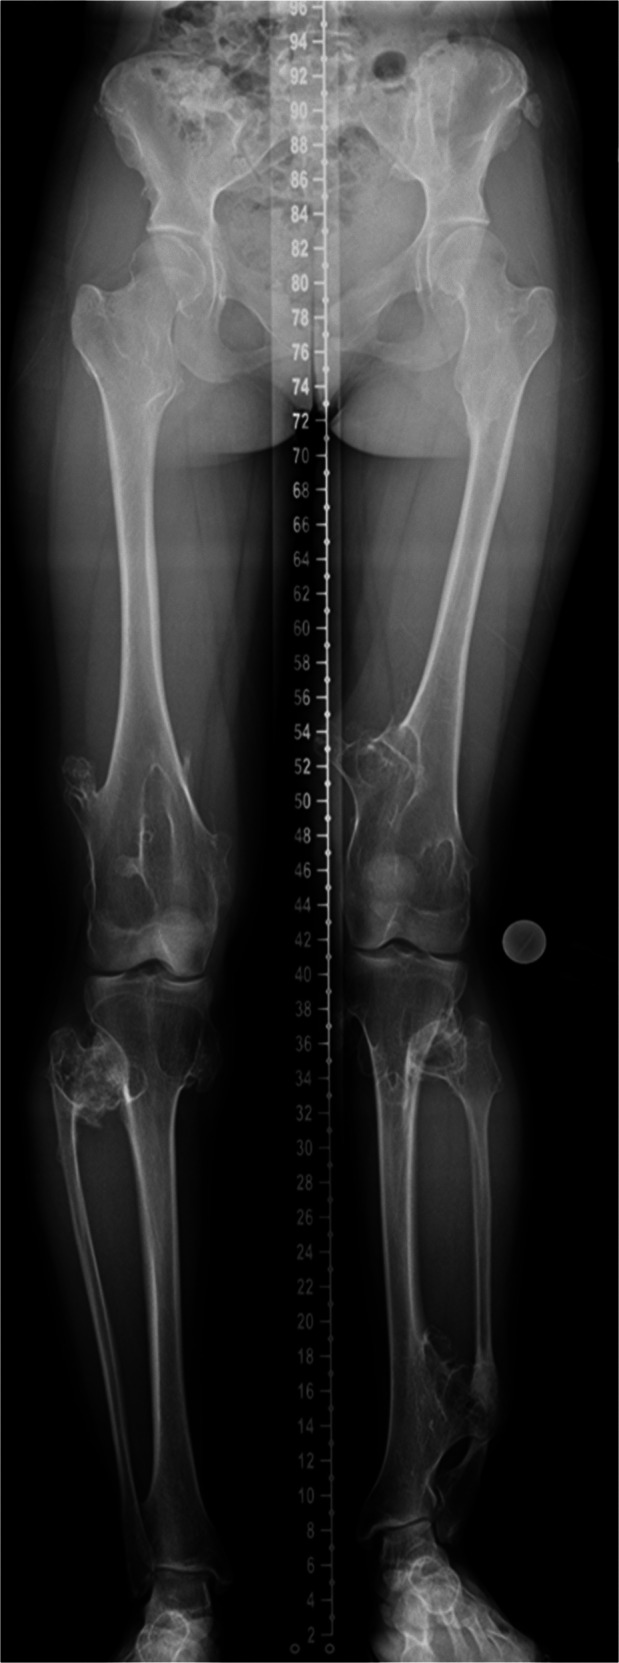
Twenty-one-year-old female with multiple hereditary exostoses. Long leg radiograph (with 3-cm block under the left leg) reveals multiple sessile and pedunculated osteochondromata, with evidence of osseous remodelling including metaphyseal widening, osseous bowing and growth disturbance with leg length discrepancy
